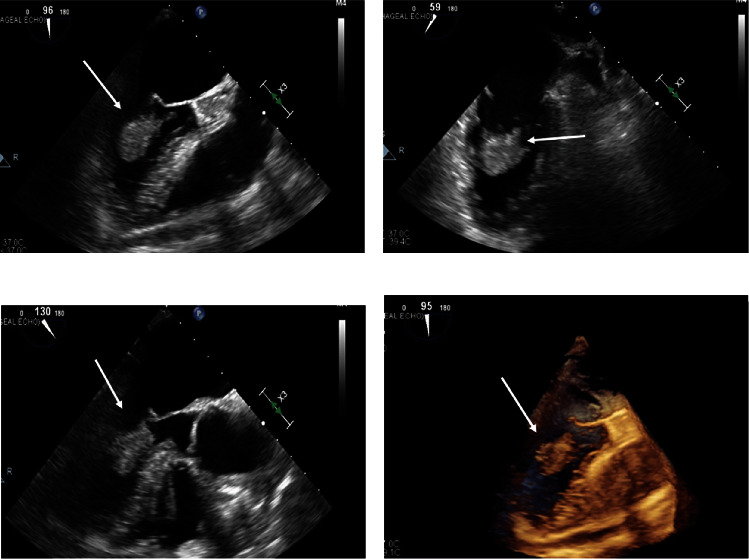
Transesophageal echocardiogram. Panels (a), (b), (c), and (d) show a large echogenic mass attached to the mitral valve apparatus and interventricular septum at different orthogonal views.
